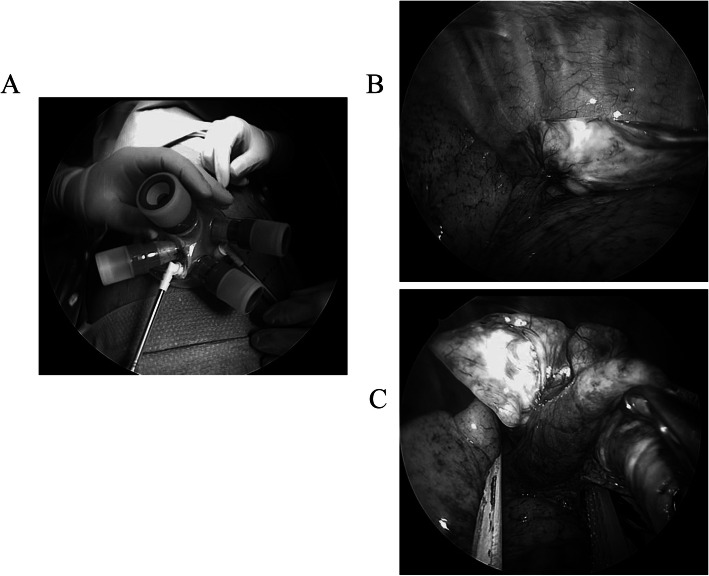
Intraoperative findings. a A 3.0 cm skin incision was made, and the Lap-protector (alnote LapSingleTM, Applied Alfresa Pharma Coroperation, Japan) was then placed through the incision. b A pedunculated tumor protruded into the thoracic cavity from the visceral pleura, and was attached to the left lower lobe. c The tumor stalk was resected using an articulating endostapler (Covidien, Norwalk, CT, USA)
